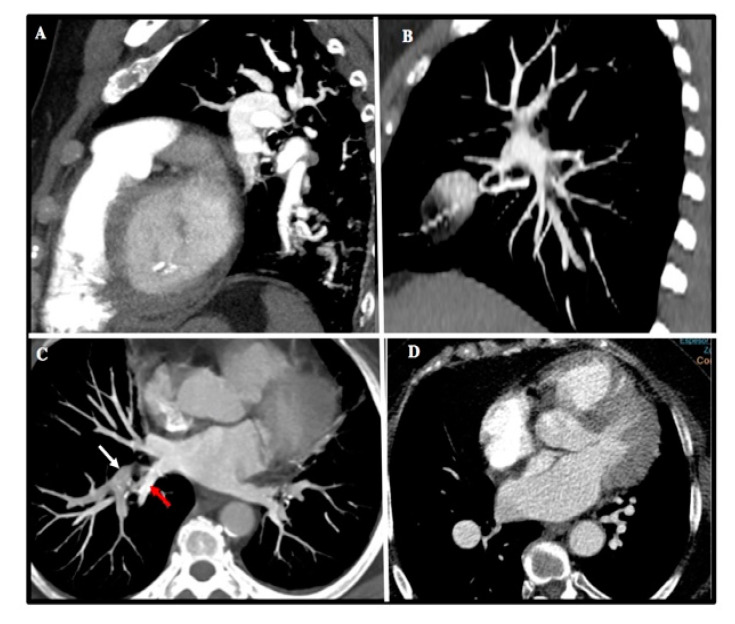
Common imagen artifacts found in MCTA. (A) Motion artifact at lower lobes. (B) CT stair artifact due to inadequate slice thickness. (C) Suboptimal contrast opacification of right upper lobe artery (white arrow) and inferior right vein (red arrow). (D) CT image noise in an obese patient.
